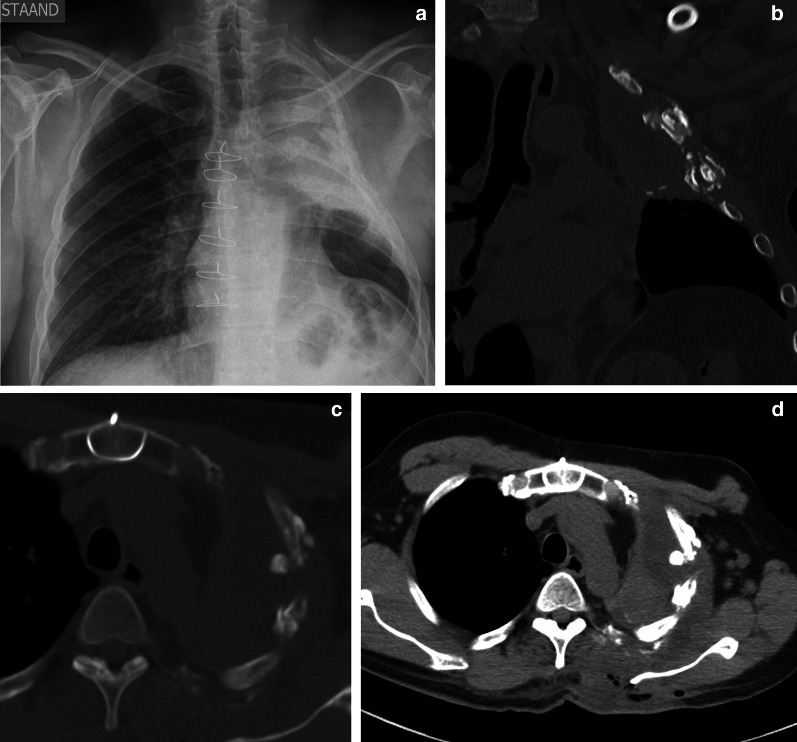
Diagnosis: radio-osteonecrosis and/or osteomyelitis. Technique: standard chest radiography and contrast-enhanced chest CT. Description: A 43-year-old man presented with left-sided chest pain. His medical history includes a lobectomy of the left upper lobe and radiotherapy. The postero-anterior chest radiograph (a) shows deformity and volume loss of the left hemithorax with a dense aspect of the lobectomy space. The coronal and axial CT thorax in bone window (b, c) reveals extensive periosteal reaction of the left ribs with inhomogeneous osteosclerosis and necrotic bone (with a typical denser aspect). The mediastinal window setting (d) is better to evaluate the adjacent soft tissue component and the fluid in the lobectomy space
